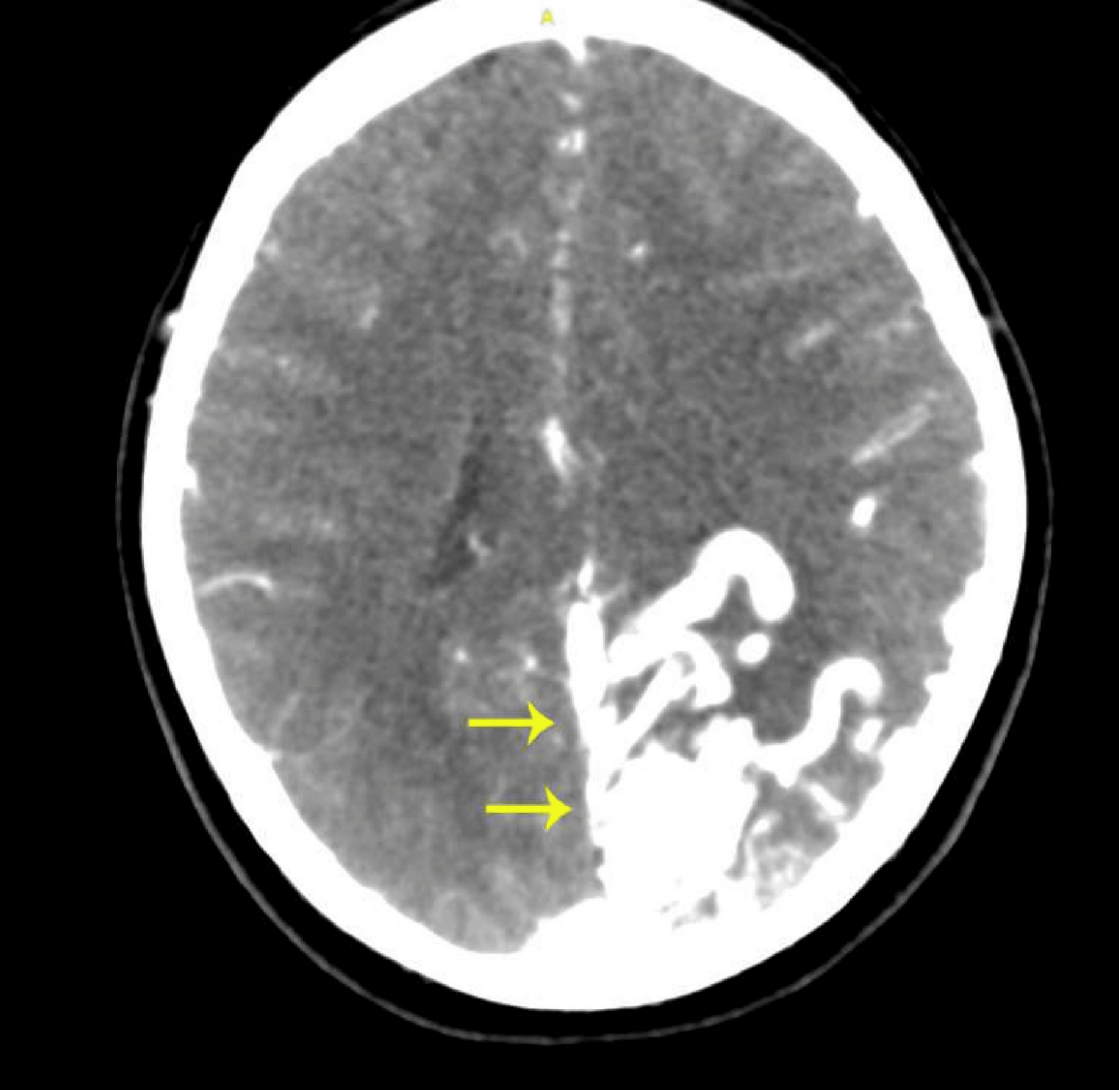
Computed tomography angiography (CTA) of the head showing a large, complex arteriovenous malformation measuring approximately 4 cm, supplied by branches of the left middle and posterior cerebral arteries, with venous drainage into the superior sagittal sinus.
